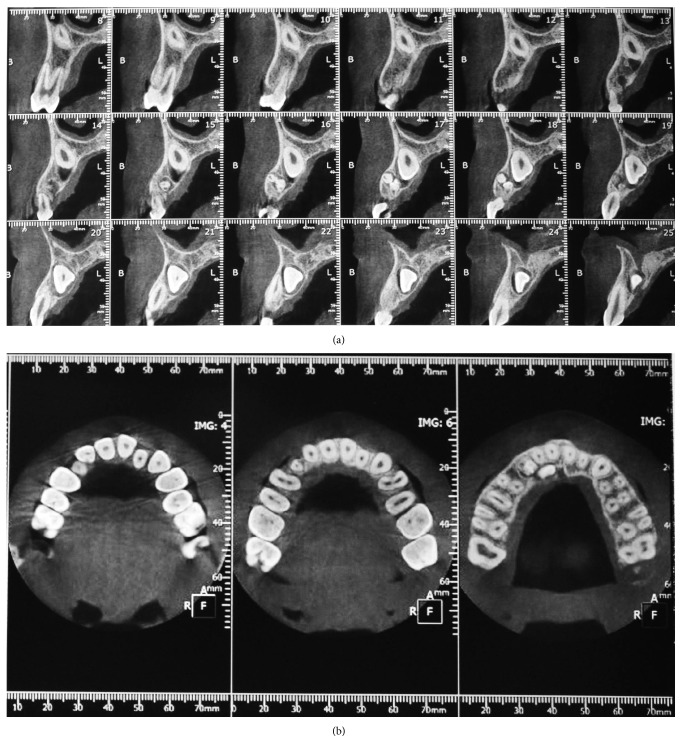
CT Dentascan: sagittal and axial view.
